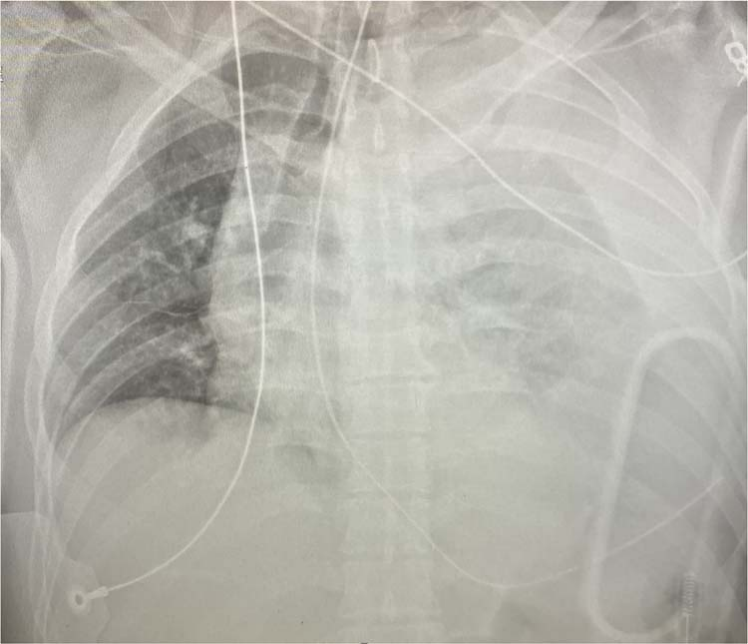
Chest x-ray demonstrating left hemothorax.
